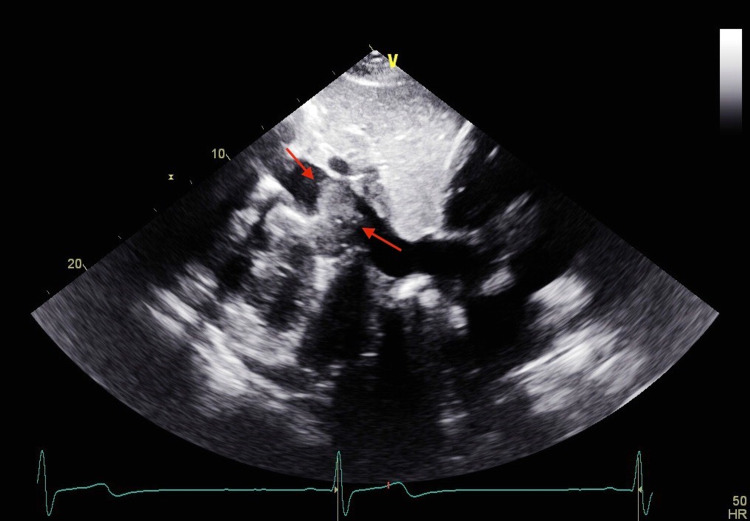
Cystic mass of 2.8 x 3.2 cm compressing the inferior vena cava on ECHO. ECHO: echocardiogram
